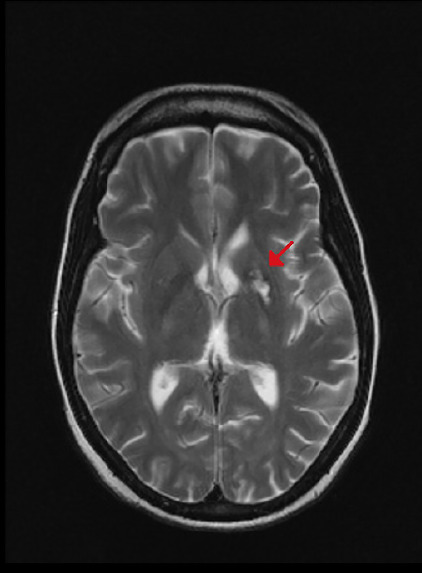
MRI of the brain shows a residual lesion in the left nucleocapsular region (red arrow).
